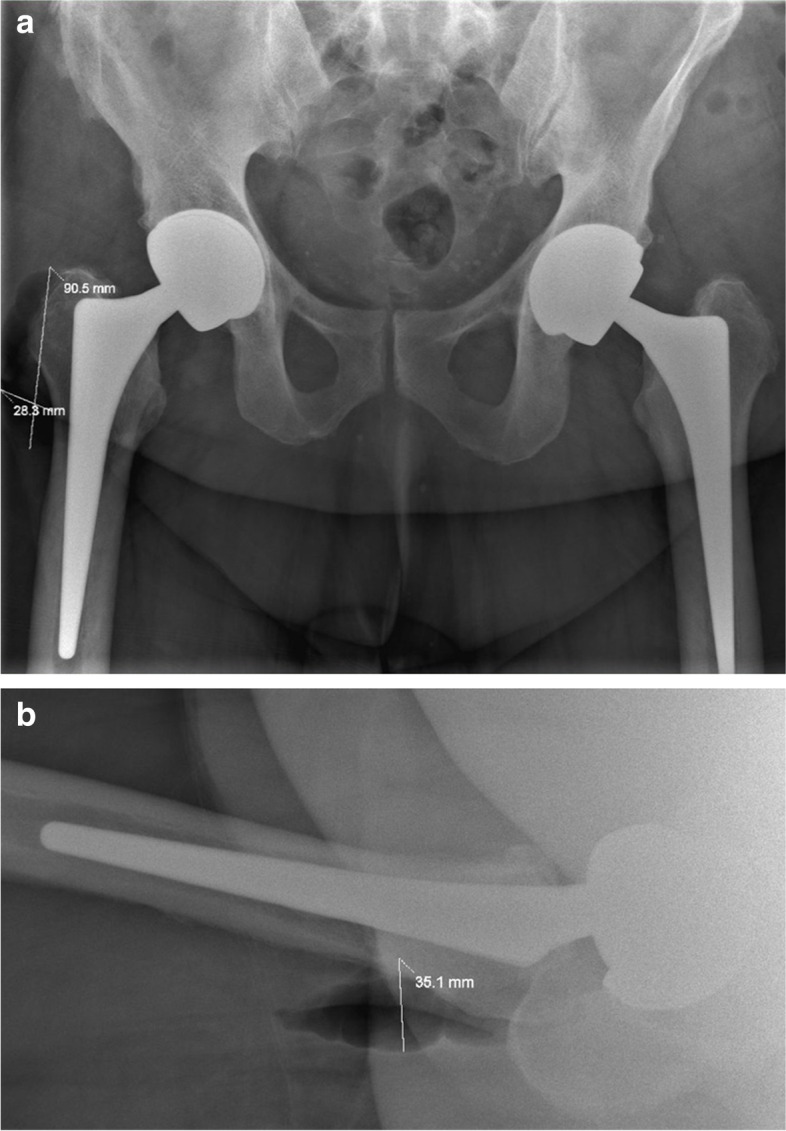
AP pelvis (a) and lateral (b) radiographs showing periarticular gas and suspicion of ceramic liner disimpaction on the right THA
